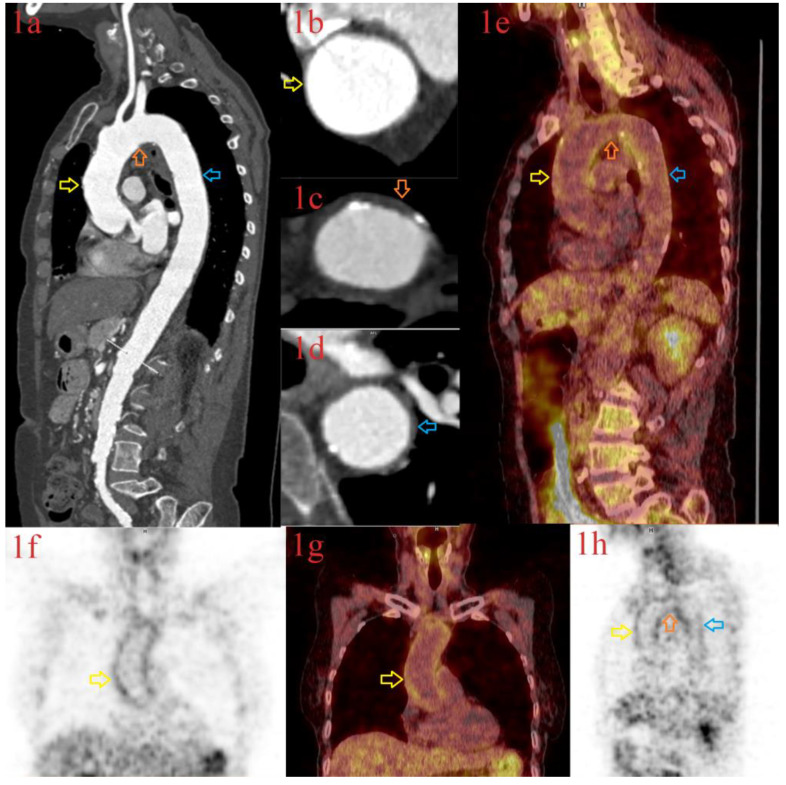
CT aortography (Images (1a–1d)) and Whole-body FDG PET-CT (Images (1e–1h)) in a 63-year-old female with large-vessel vasculitis showing inflammation of the aortic wall at the corresponding levels on the following localization: the ascending aorta (CT: Image (1a,1b); PET-CT: Image (1e–1h); red arrows), the aortic arch (CT: Image (1a,1c); PET-CT: Image (1e,1h); orange arrows) and the descending thoracic aorta (CT: Image (1a,1d); PET-CT: Image (1e,1h); blue arrows).
